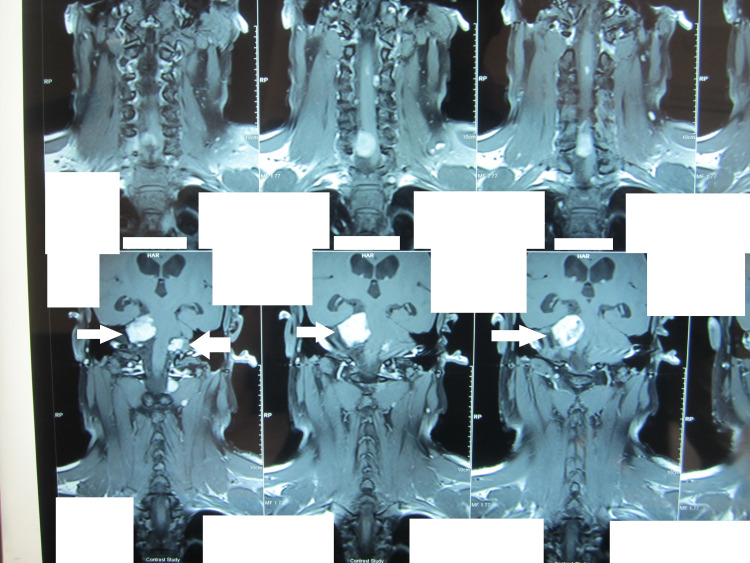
MRI brain/cervical spine coronal section without contrast in T2 sequence showing bilateral vestibular schwannomas (arrows) MRI: magnetic resonance imaging
